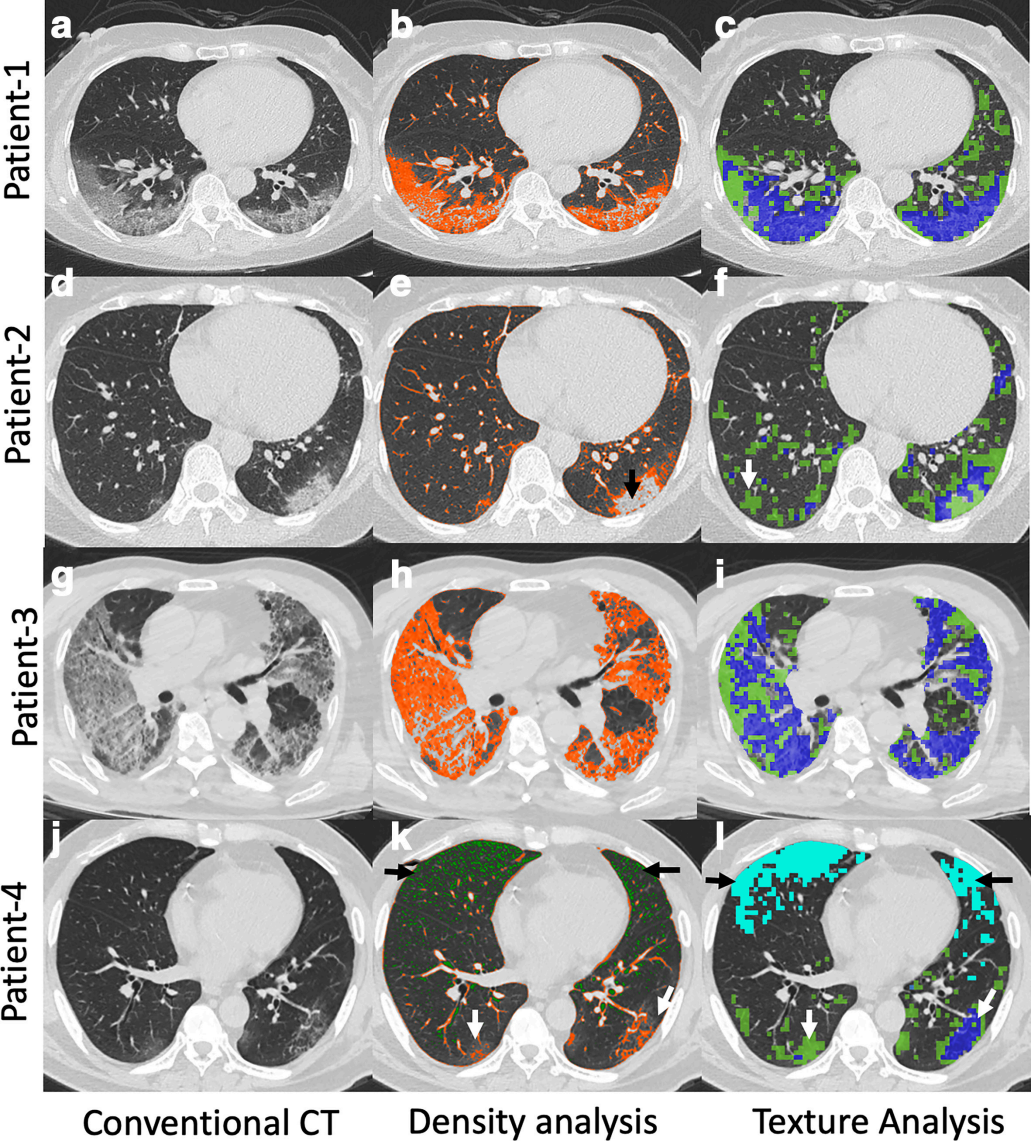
Conventional CT (first row), lung density (second row), and 3D AMFM Texture (third row) quantification in four patients with COVID-19 pneumonia. Patient 1: 67-year-old female with conventional CT (a) demonstrating posterior bilateral lower lobe mixed GGO and interstitial thickening. Density analysis (b) demonstrating HAAs (orange color) which was quantified as 14%. 3D AMFM Texture analysis (c) demonstrating abnormal lung (green color = GGO; blue color = GGO with reticular thickening) which was quantified as 27% (GGO,16%; GGO with reticular thickening, 11%). Patient 2: 46-year-female with conventional CT (d) demonstrating left more than right high-attenuation posterior GGO. Density analysis (e) underestimated the disease (quantified as 8%) because of relatively high attenuation in the central portion of the abnormality (black arrow). However, 3D AMFM Texture analysis (f) quantified the entire extent of disease, characterized it as predominantly GGO (green, 15%) with intermixed areas of interstitial thickening (blue, 3%), and also highlighted certain areas in the right lung (white arrow) that did not have identifiable disease on conventional CT. Patient 3: Adult male patient with COVID-19 pneumonia, conventional CT (g) demonstrating extensive bilateral disease with intermixed areas of GGO and interstitial thickening. Density analysis (h) showing HAA (orange color) quantified as 46% and 3D AMFM Texture analysis (i) quantifying the amount of GGO (green color, 31%) and mixed GGO and interstitial thickening (Blue, 35%). Patient 4: Conventional CT (j) demonstrating bilateral lower lobe GGO intermixed with areas of interstitial thickening with disease more in left. No abnormality is appreciated in the anterior lung on conventional CT. Density analysis (k) showing HAA (orange, white arrows) quantified as 6% with low attenuation areas in the anterior lung (green color in K, black arrows, 5%). 3D AMFM Texture analysis (l) also demonstrated abnormality corresponding to a region of hyperlucency (light blue, black arrows, 10%) in the anterior lung with better quantification of disease (GGO 8%, GGO with reticular thickening 2%) in the posterior lungs as compared to density analysis (white arrows in L). hyperlucent areas, which visually do not appear to be emphysematous, (detected as a texture abnormality) are possibly due to microemboli-induced reduction in regional blood volume. Despite relatively less lung involvement, this patient had a protracted ICU course and died on Day 25. AMFM, adaptive multiplefeature method; GGO, ground glassopacity; HAA, high attenuation area.
